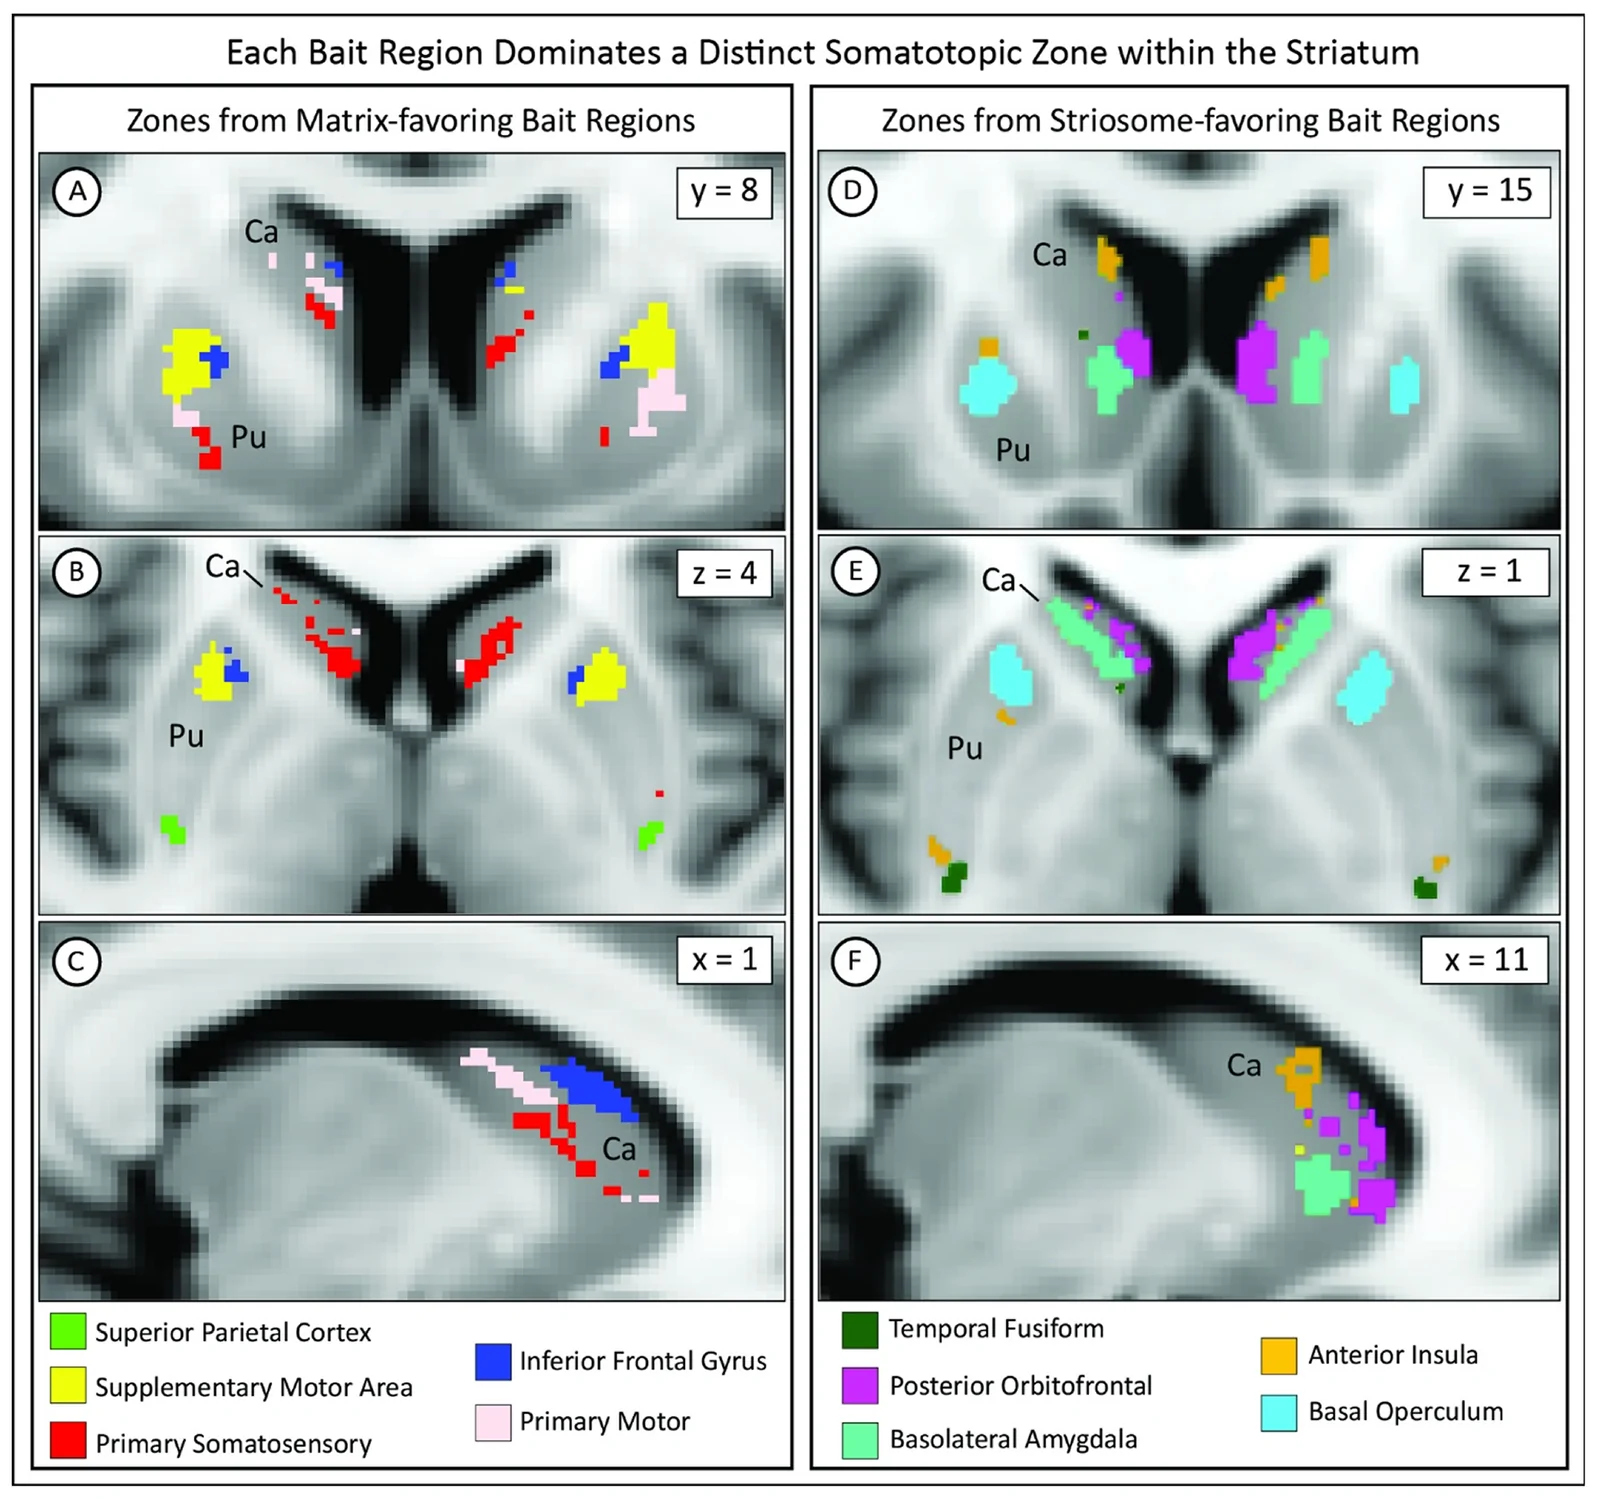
While each bait region can influence compartment bias throughout the striatum, each region dominated compartment-like bias in a distinct somatotopic zone, as seen in the coronal (A, D), axial (B, E), and sagittal (C, F) planes. Zones influenced by matrix-favoring bait regions are shown in (A–C), while zones influenced by striosome-favoring bait regions are shown in (D–F). Zones might share a border but never overlapped. Although we parcellated the left and right hemispheres independently, the location of each somatotopic zone was highly similar between hemispheres. All zones had equal volume. Pu, putamen; Ca, caudate. The images adhere to radiographic convention. Coordinates follow MNI convention.
